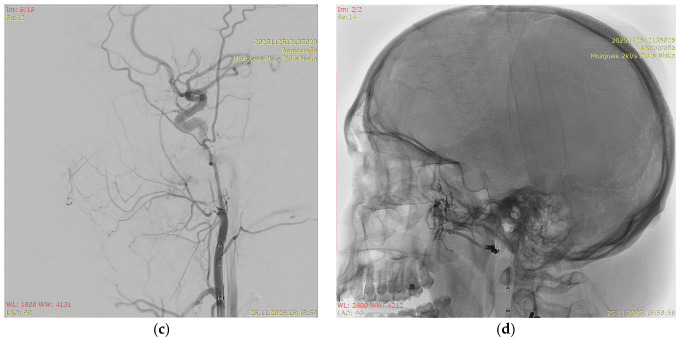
Skull-base arteriovenous malformation (AVM) in Patient Y. (a) Pre-treatment image of the malformation; (b) microarteriography of the lesion; (c) post-treatment vascular image; (d) X-ray image demonstrating the embolic material. The term “mózgowe 2 kL/s, 2 kL/s niska” is translated as “Cerebral 2 fps, 2 fps Low”.
